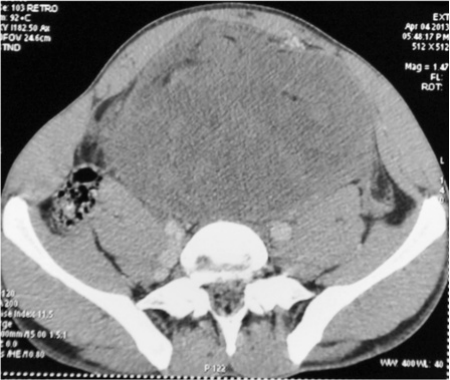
Abdomino-pelvic computed tomography showed a massive, inhomogeneous, hypodense abdominal tumor.
